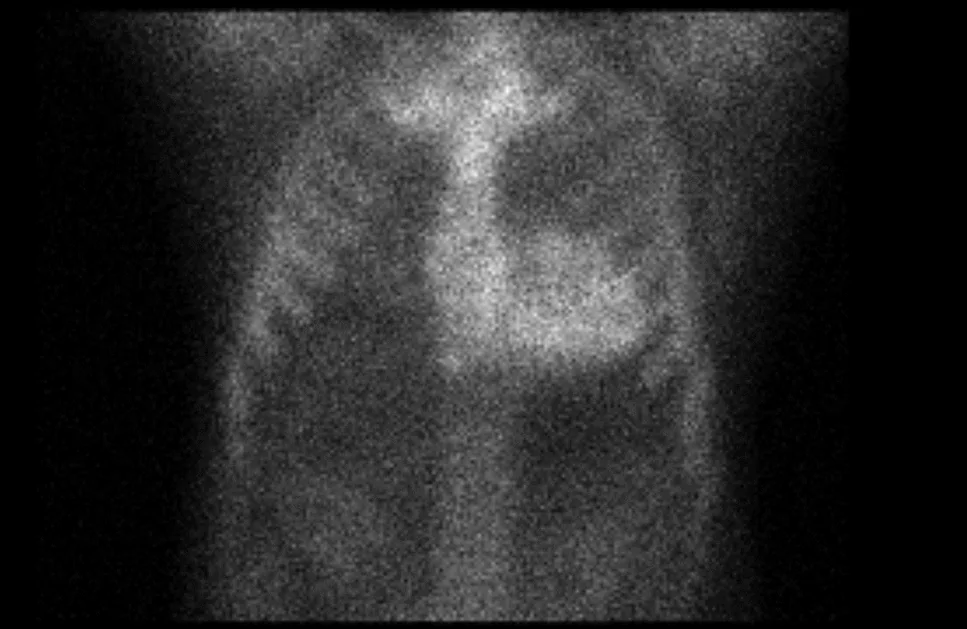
99mTc-3,3-diphosphono-1,2-propanodicarboxylic acid scintigraphy was conducted, showing a cardiac uptake compatible with a Perugini score of 2
